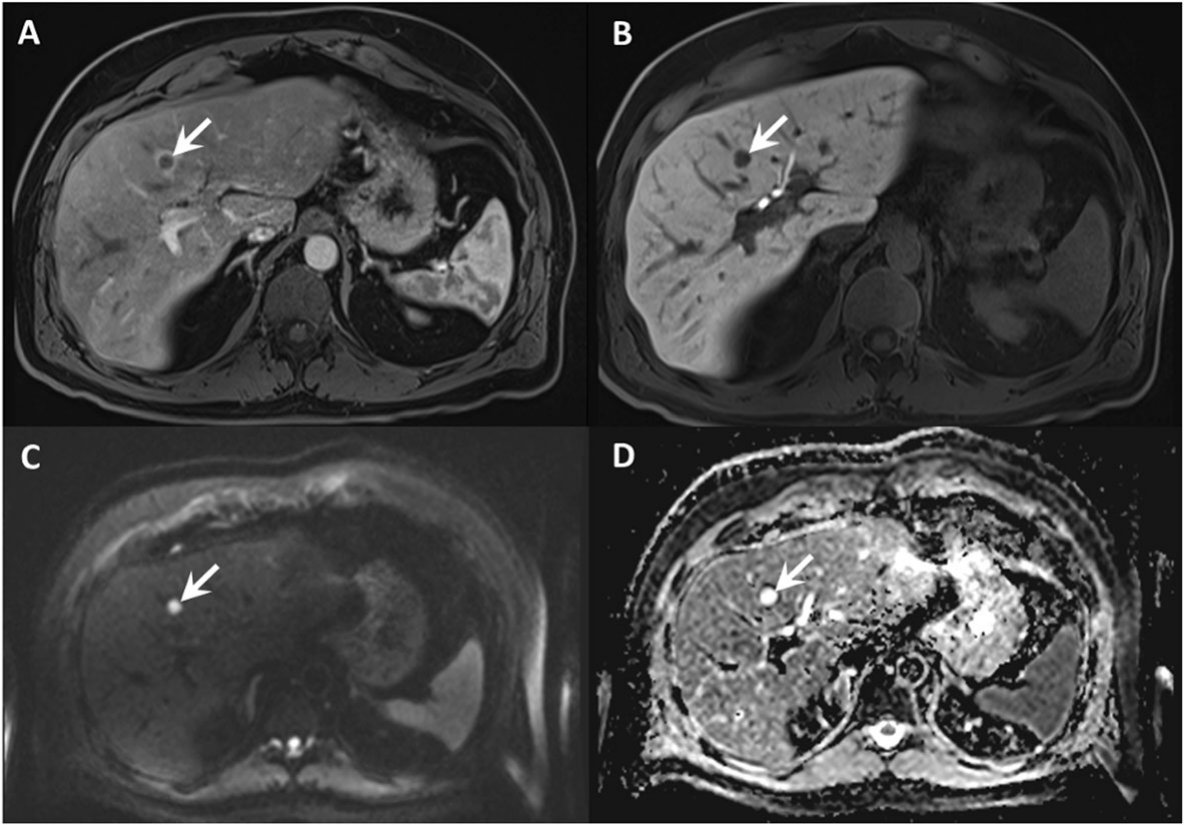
Lesion detection on contrast enhanced images compared to DWI and ADC maps. a, b T1 GRE fs with arterial and hepatobiliary phase. c, d DWI, b800 and ADC map. Contrast enhanced T1 images show a centrally hypointense lesion (arrow) with a hyperenhancing rim in the arterial phase and no contrast uptake in the hepatobiliary phase, indicating a partially cystic metastasis (a and b). Note the T2-shine through on DWI due to its cystic character (c and d)
